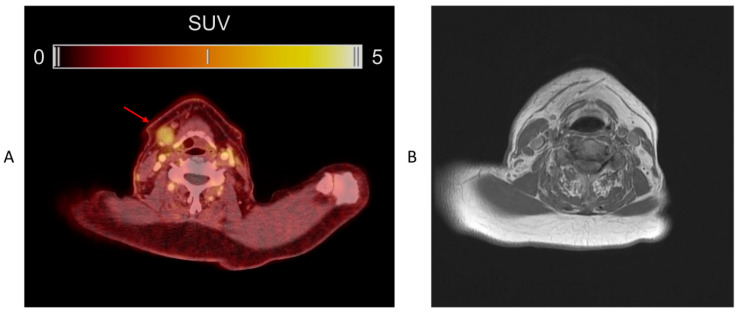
(A) ^68^Ga-NOTA-AE105 uPAR-PET/CT of a patient with lymph node metastasis, not detected by routine imaging work-up (CT/MRI). (B) MRI from the same patient.
